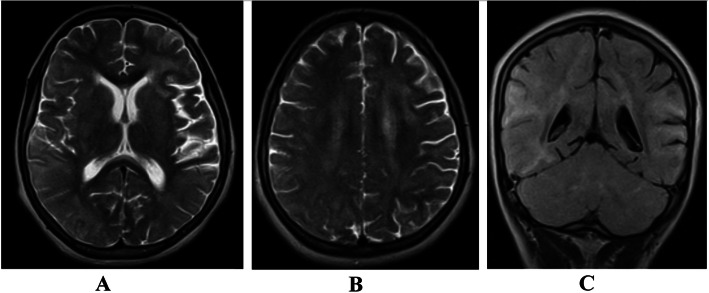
a, b Axial MRI showing diffuses both cortical and white matter T2 hyperintense lesions without mass effect (a, b). c Coronal FLAIR MRI sequence, showing hyperintense lesions on cortical and white matter areas of brain without mass effect
